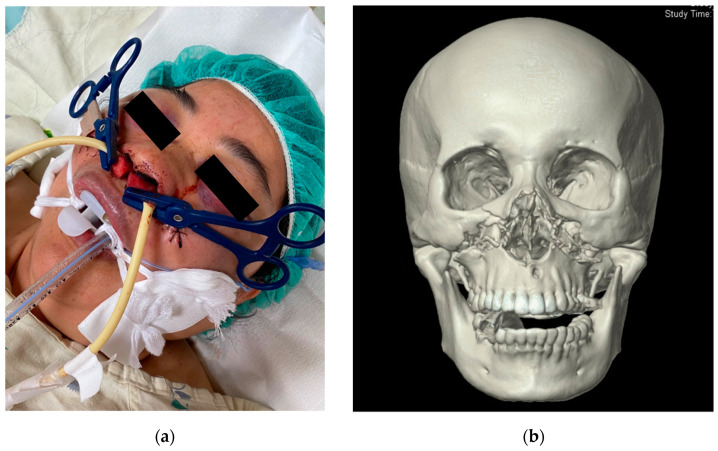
A 55-year-old female who presented with massive oronasal bleeding after facial trauma. (a) Patient was intubated for airway protection. Arterial and posterior nasal packing was performed after intubation. (b) CT scan shows bilateral maxillary fractures.
